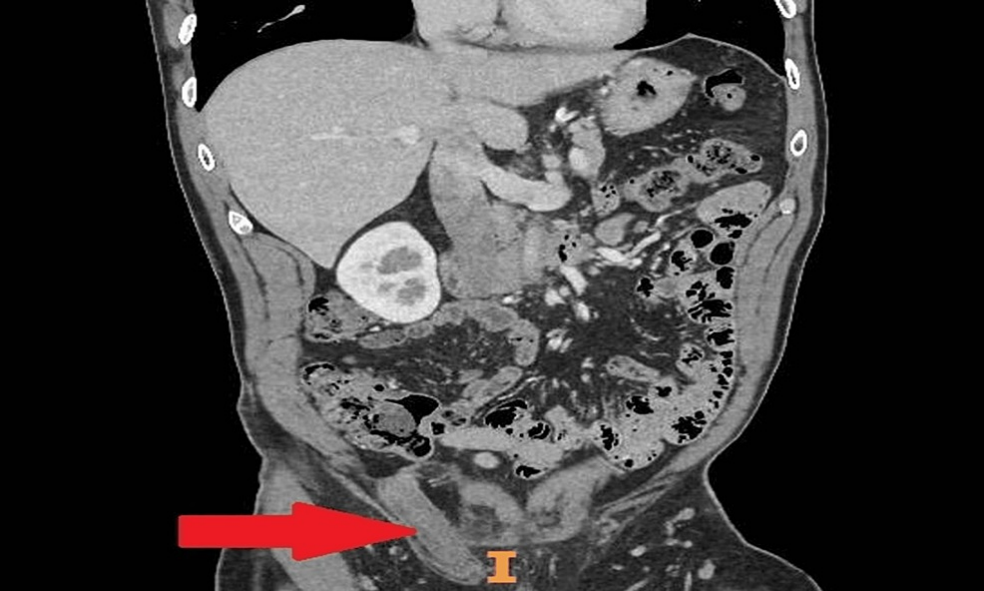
A coronal view of a CT scan of the abdomen and pelvis with contrast The scan shows thickening and inflammatory changes associated with the inguinal canal likely secondary to inflammation of the contained sperm.
